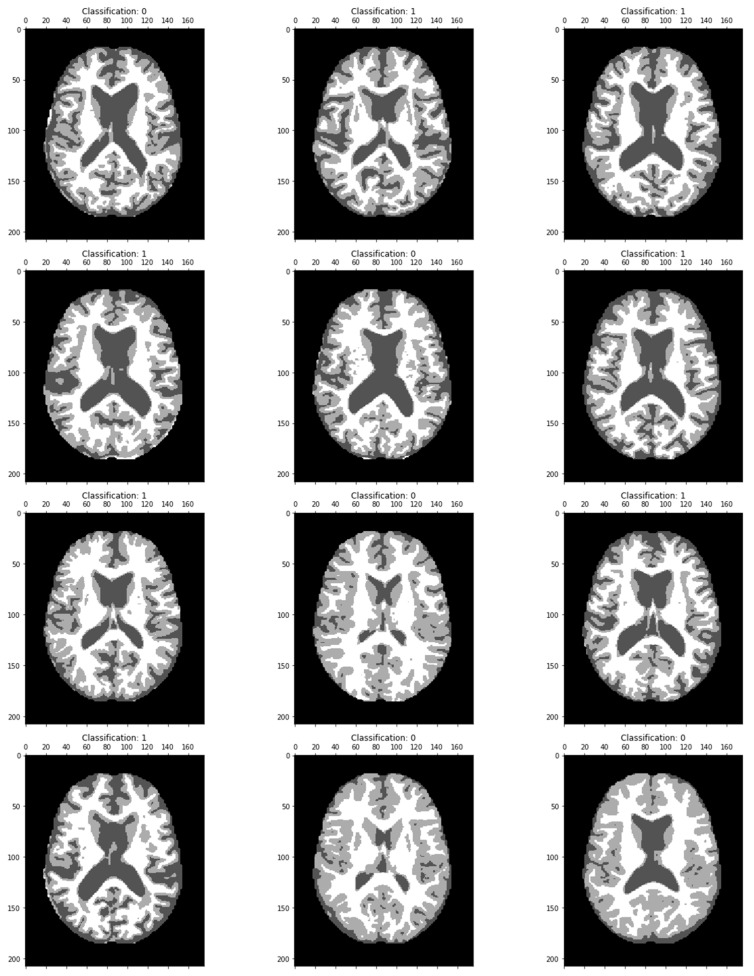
MRI image classification results showing the classification value as 0, confirming the absence of Alzheimer’s disease, and Classification 1 shows the brain image of an Alzheimer-affected person.
